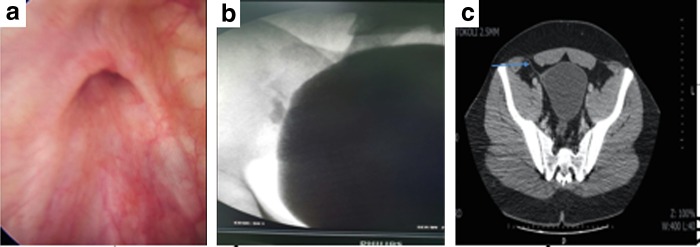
(a) Cystoscopic image of appendicovesical fistula (AVF) in right lateral wall of the bladder dome. (b) Contrast agent near the right side of the bladder in voiding cystography. (c) Detected a fistula tract between the distal end of the appendix and right lateral wall of the bladder dome in CT scan.
